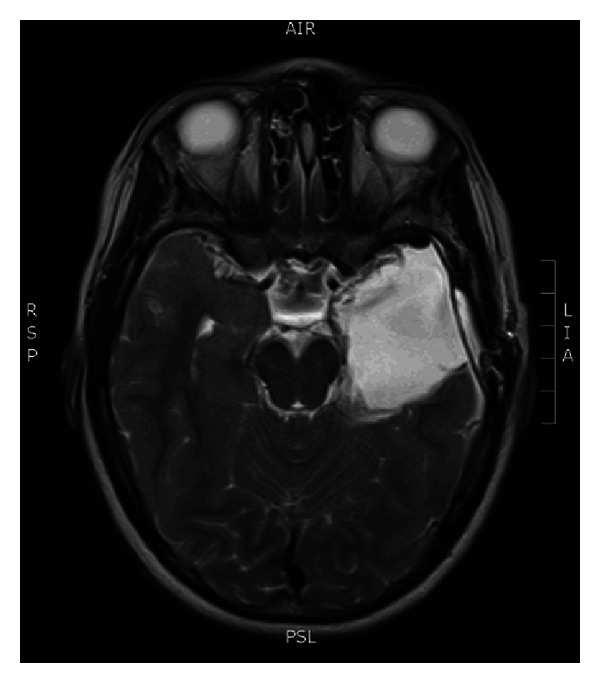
Postoperative axial T2-WI MRI scan at the same level after left temporal lobe resection.
